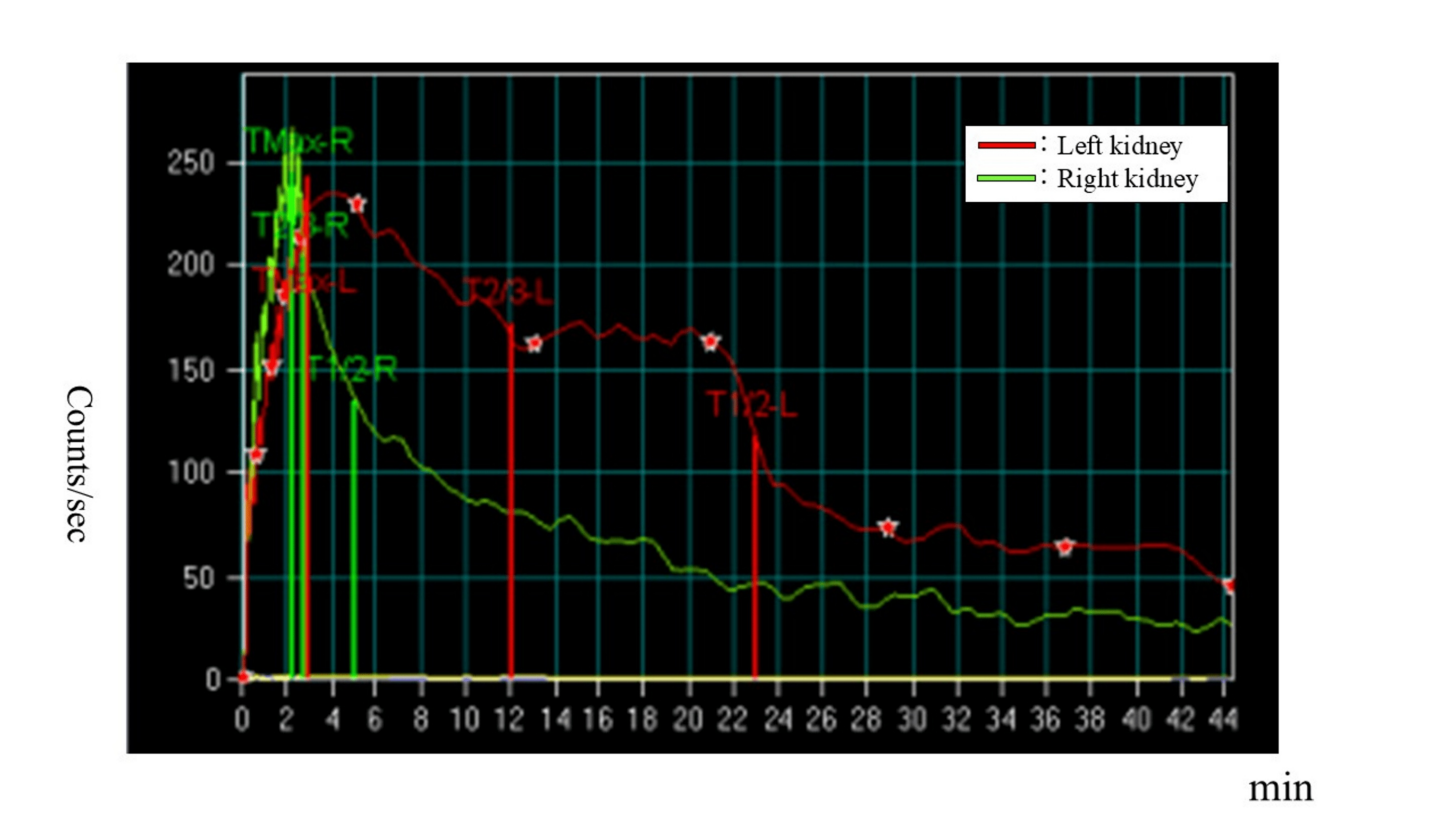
Mercaptoacetyltriglycine (MAG3) scintigraphy at eight months of age. Differential renal function (DRF) was preserved (42.6% left, 57.4% right). The red line is on the left kidney. The green line is on the right kidney.
